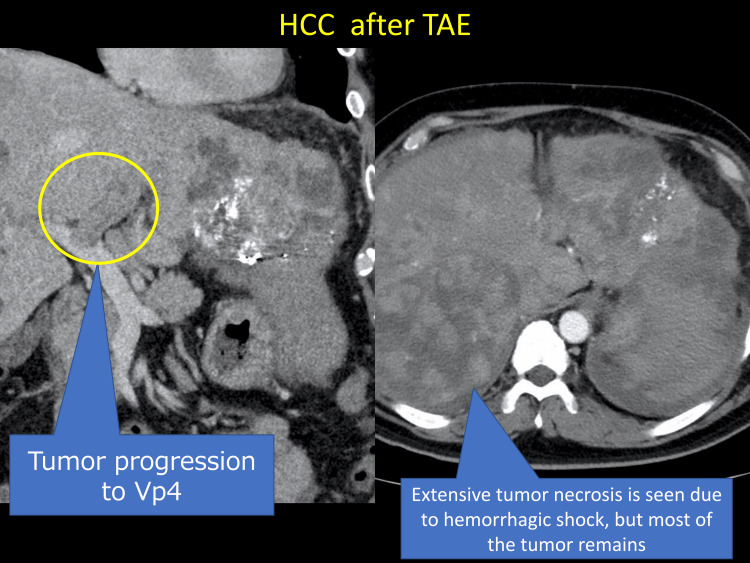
Contrast-enhanced CT after TAE for ruptured HCC. HCC: hepatocellular carcinoma; TAE: transarterial embolization
